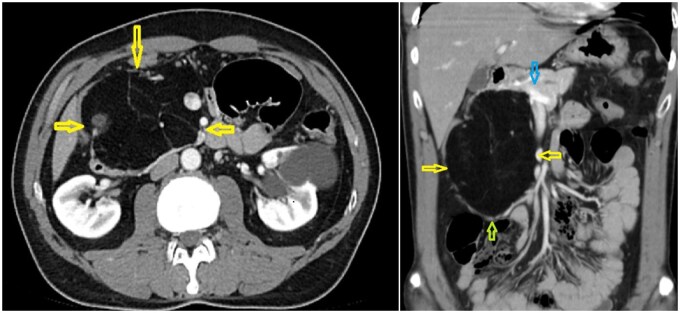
Contrast-enhanced axial and coronal CT images of a 60-year-old man with abdominal pain and vomiting demonstrate a well-defined, homogeneous, fat-attenuation mass centered in the pancreatic head. The lesion is most suspicious for a liposarcoma, supported primarily by its large size, which is a typical feature of well-differentiated liposarcomas. However, the imaging features including homogeneous macroscopic fat composition, lack of internal soft tissue nodules or post-contrast enhancement, and smooth interface with the pancreatic parenchyma are compatible with both pancreatic lipoma and well differentiated liposarcoma. Yellow arrows denote the sharply marginated, encapsulated fat-density mass in the pancreatic head. The blue arrow indicates the pancreas. The green arrow shows adjacent small-bowel loops displaced by mass effect.
